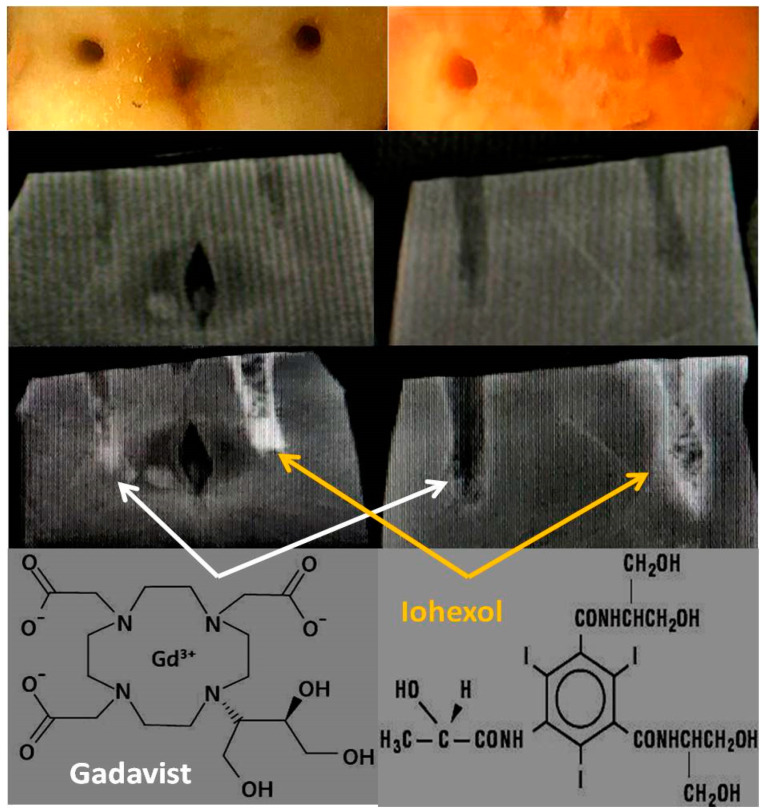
X-ray images of the mineral-rich model fruits and vegetables. 1st row: Cut samples: apple (left) and sweet potato (right) and manually carved holes (2 × 2 × 10 mm^3^ or 0.04 mL for apple and approx 3.3 × 3.3 × 20 mm^3^ or 0.2 mL for sweet potato). 2nd row: Pre-contrast X-ray images at 44 keV/15 mAs by a clinical scanner. 3rd row: Post-contrast X-ray images showing Gadavist is perfused in the left wells (white arrows) and Iohexol in the right wells (yellow arrows) for both fruits. 4th row: Molecular structures of both of the contrast media.
